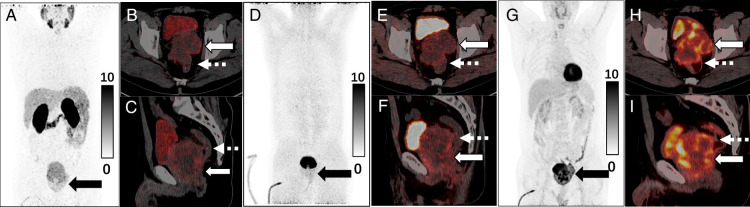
Multi-tracer PET/CT imaging was performed for preoperative systemic evaluation (Ethics No.: YX-2021-113-01). PET/CT maximum intensity projection (MIP, A) and axial (B)/ sagittal (C) images showed moderate ^68^Ga-PSMA uptake of the prostate lesion (SUV_max_: 7.17). Recognizing the “FAPI-high/FDG-low” imaging signature of mucinous adenocarcinomas, ^68^Ga-FAPI and ^18^F-FDG PET/CT were conducted. The lesion demonstrated moderate ^68^Ga-FAPI (D, MIP; E and F, PET/CT) uptake (SUV_max_: 12.75) and apparently higher ^18^F-FDG (G, MIP; H and I, PET/CT) uptake (SUV_max_: 25.46). Images revealed anterior rectal wall infiltration (dashed arrows) and confirmed the prostate lesion as the primary malignancy due to no extra-prostatic abnormal uptake. The marked FDG avidity, compared with PSMA and FAPI uptake, suggests a unique pathologic subtype of prostate cancer.
